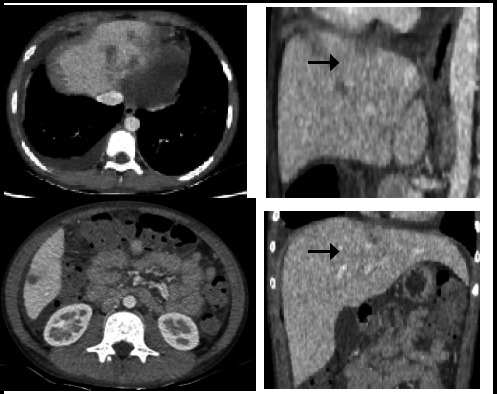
CT chest and abdomen showing right pleural effusion with pericardial effusion, ascites, and infiltrates in liver.
